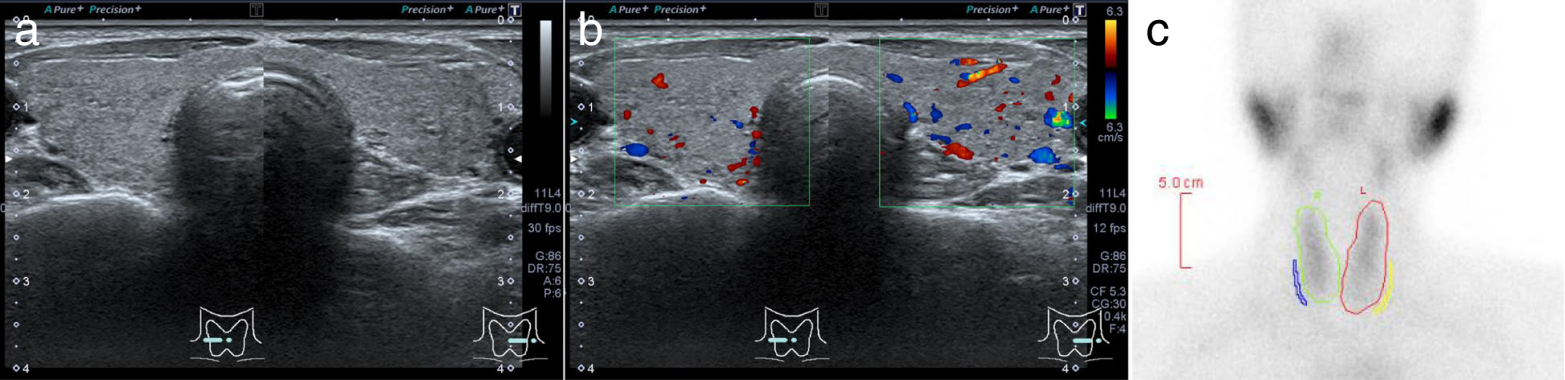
Thyroid gland imaging findings (July 2017). a, b Ultrasonography of the thyroid gland showing rough and mildly low echogenicity in a slightly enlarged thyroid gland without a tumor (a). Color Doppler images revealed no increased blood flow in the thyroid gland (b). c Technetium-99m pertechnetate thyroid scintigraphy showing a low thyroid uptake (0.1%, reference range 0.5–4%) in the entire thyroid gland without a hot spot
